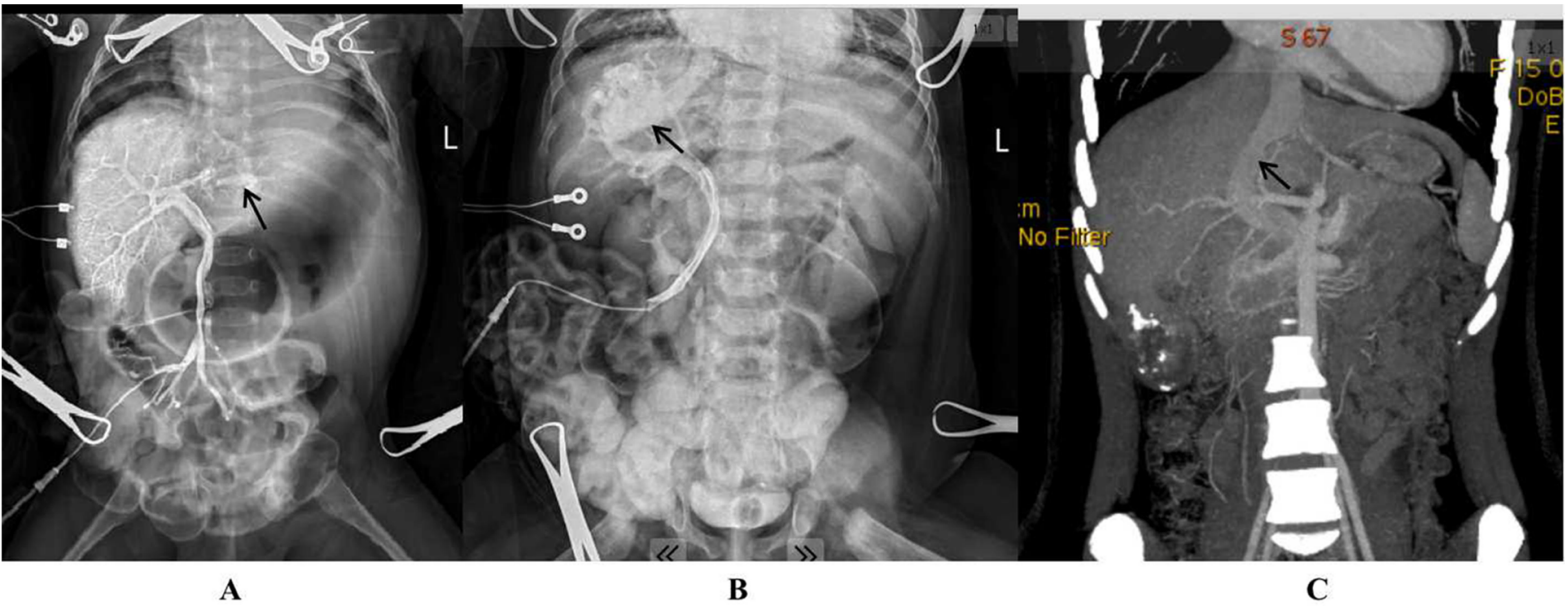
Intrahepatic portosystemic shunt (arrow) as demonstrated by intraoperative portal venography (A: a shunt from the left portal vein to the left hepatic vein; B: a shunt from the right portal vein to the right hepatic vein; C: a patent ductus venosus shown by preoperative CT three-dimensional imaging).
